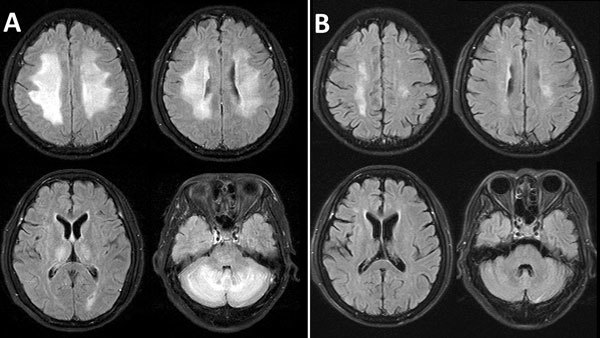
Fluid-attenuated inversion recovery magnetic resonance images of the brain of a 55-year-old woman with dengue-associated posterior reversible encephalopathy syndrome, Ho Chi Minh City, Vietnam. A) Bilateral abnormal nonenhancing, confluent high signal in the periventricular and deep cerebral white matter of the high frontal parietal area and cerebellar hemispheres, thalamus, and pons. B) Almost complete resolution of abnormal findings 7 weeks later, after treatment.
